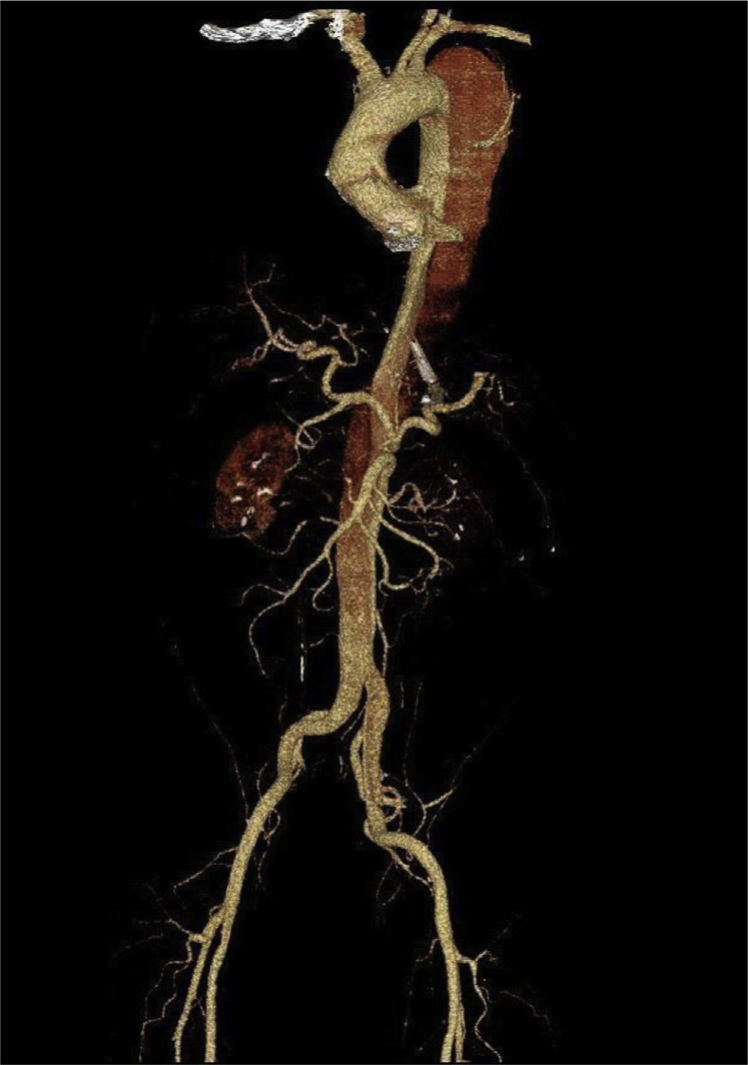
Computed tomography (CT) scan of the chest of 49-year-old man revealing an extensive type B aortic dissection (TBAD) originating in the proximal descending thoracic aorta just distal to the takeoff of the left subclavian artery. The dissection extended to involve the entirety of the abdominal aorta and both common iliac arteries. The true lumen supplied the celiac and mesenteric arteries and the left renal artery. The false lumen supplied the right renal artery. The dissection was graded as type B, 2, 10.^8^ For Type B dissections gradings can include the proximal (2 which includes the arch portion with the left subclavian origin) and distal (10 into the common illiacs) limits of the false lumen.^28^
